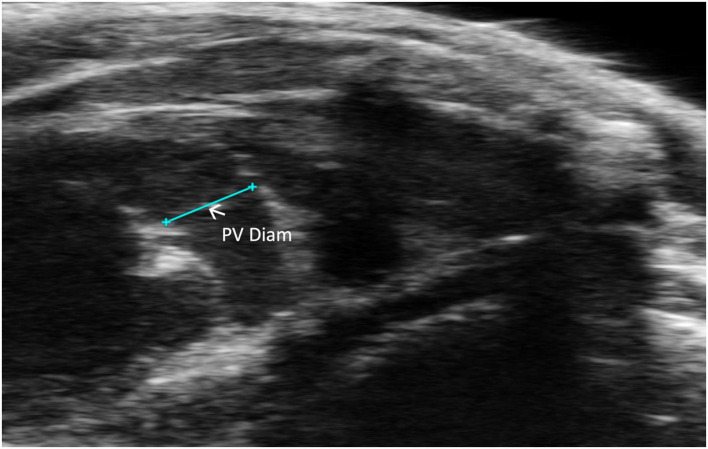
Pulmonic valve (PV) diameter is acquired and analyzed in B-mode of the parasternal long axis (PLAX) view.
